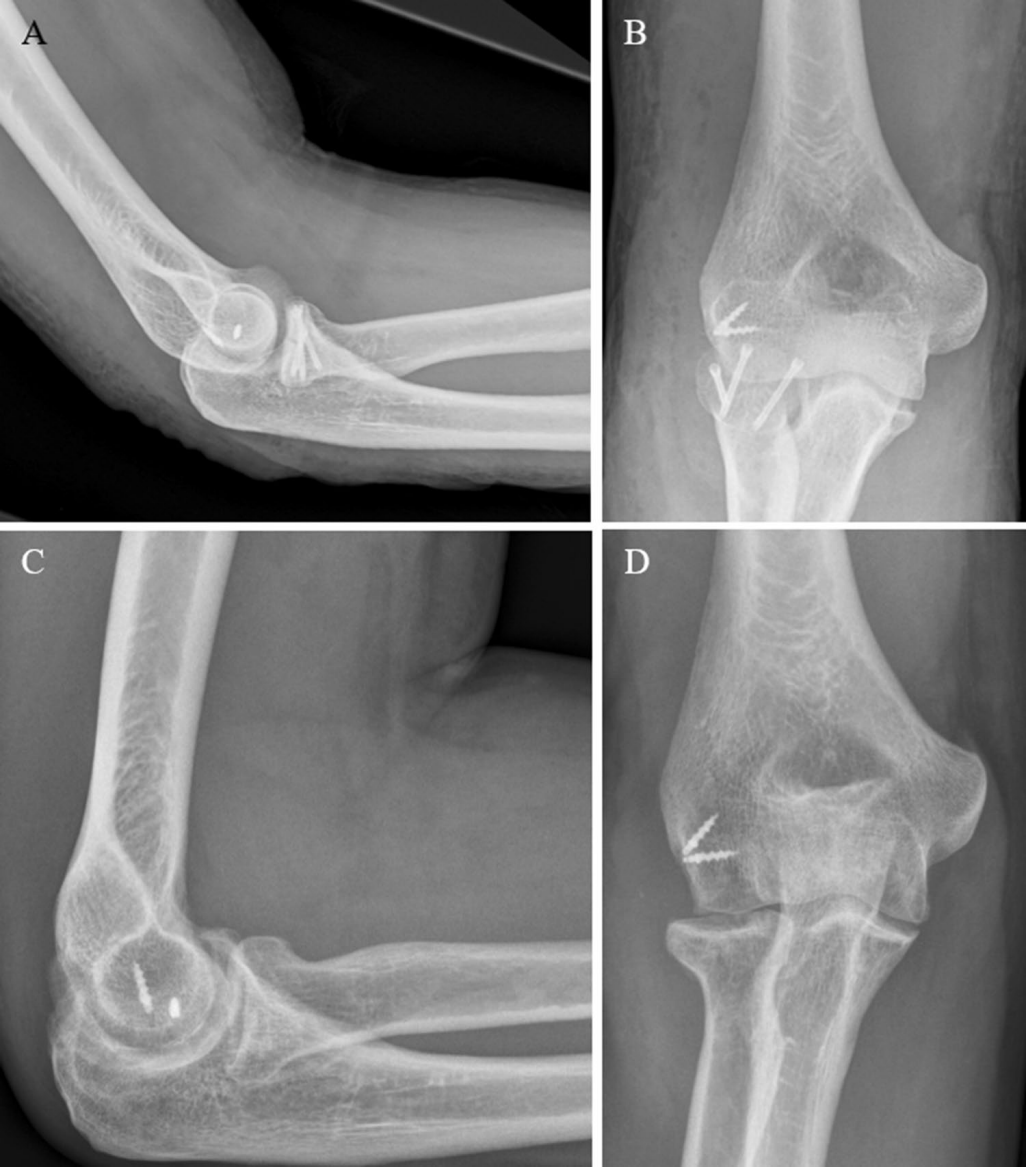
a, b Postoperative radiographs (lateral and anteroposterior) after radial head fixation with three CCS and reattached LCLC (Case 2). c and d after 6 months and metal removal
